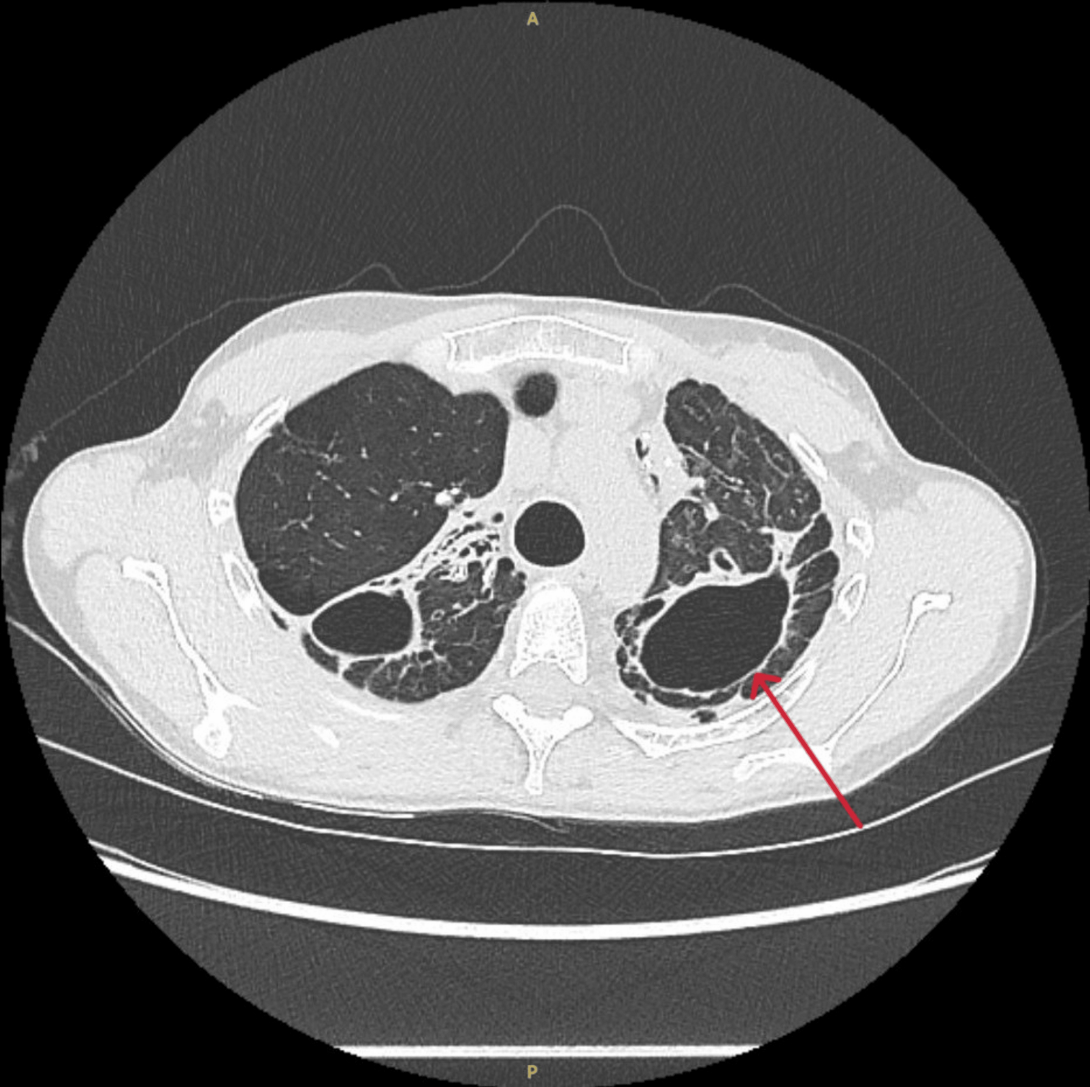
Computed tomography of the chest shows shrinkage of the abscess with a residual cavity.
